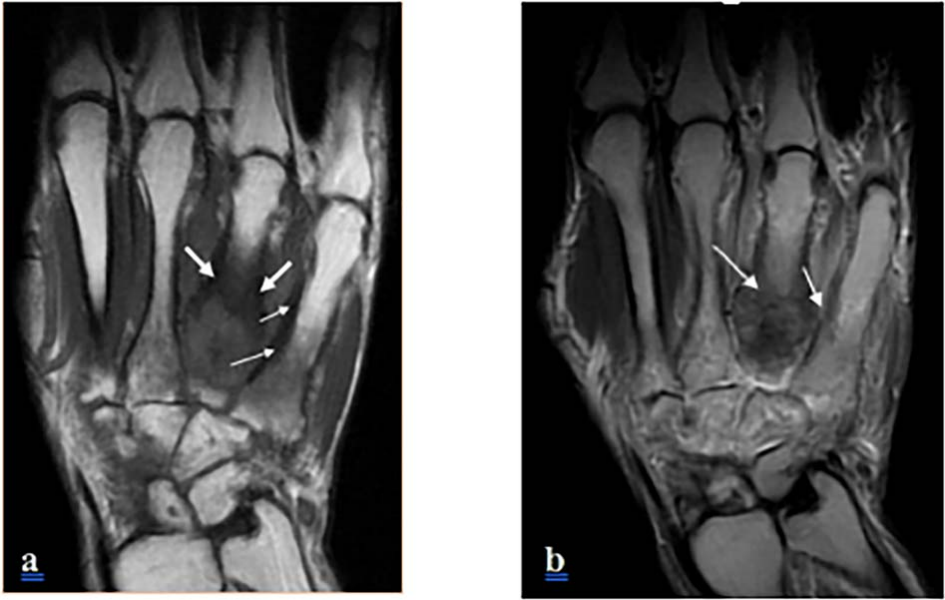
MRI of the left hand, a coronal section, on T1 (a) and T2 (b) weighted sequences, showing cortical thickening of the 4th and 5th metatarsal, as a hyposignal and isosignal on all sequences.
